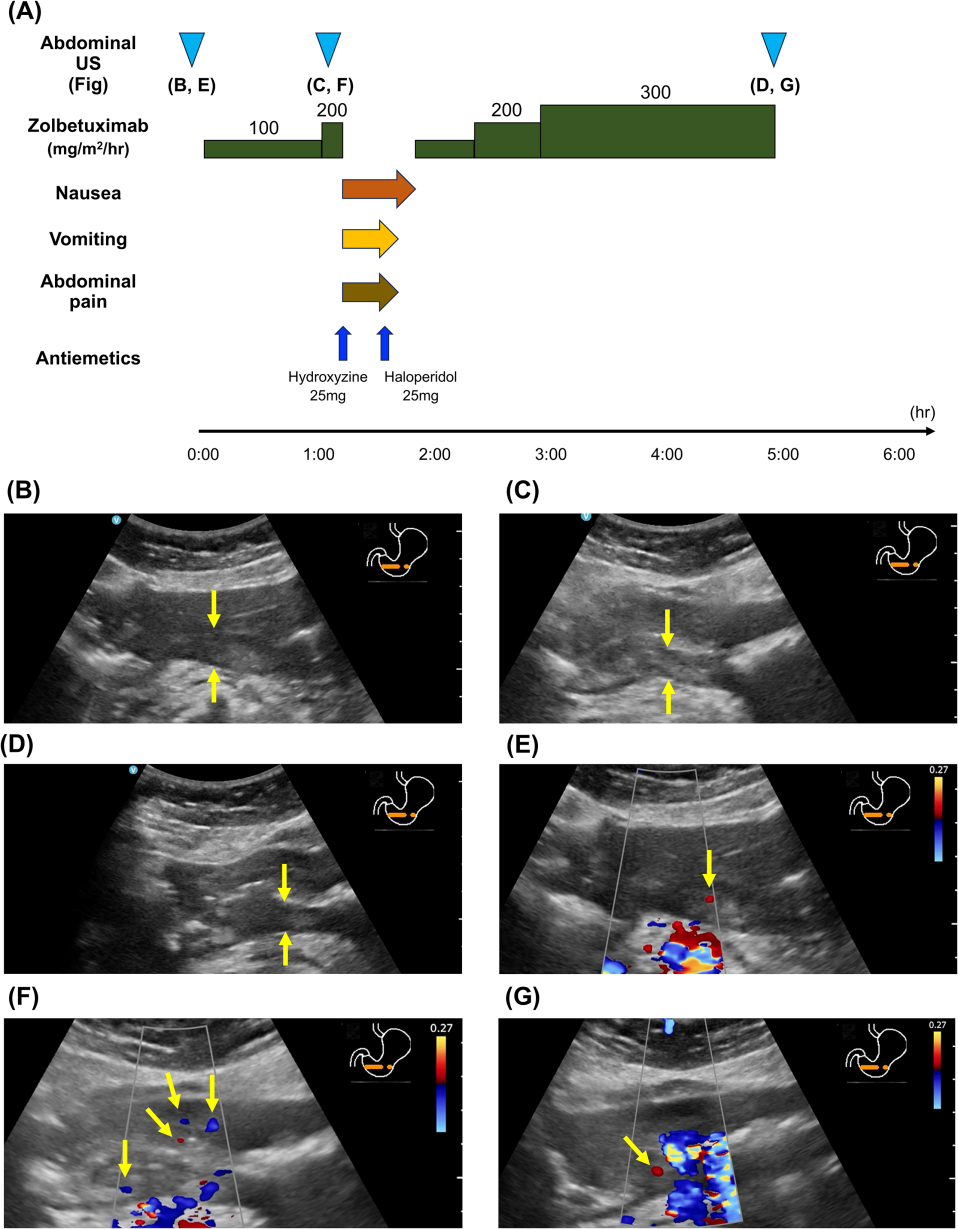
(A) Treatment course with the first cycle of zolbetuximab in Case 2. (B) At baseline, (C) after 1 h of zolbetuximab administration, and (D) after the completion of zolbetuximab administration. At the onset of nausea, increased echogenicity of the submucosal layer and enhanced layer structure were observed. Color Doppler US findings (E) at baseline, (F) at the onset of nausea, and (G) after the completion of zolbetuximab administration. An increase in blood flow signals was observed during zolbetuximab administration.
